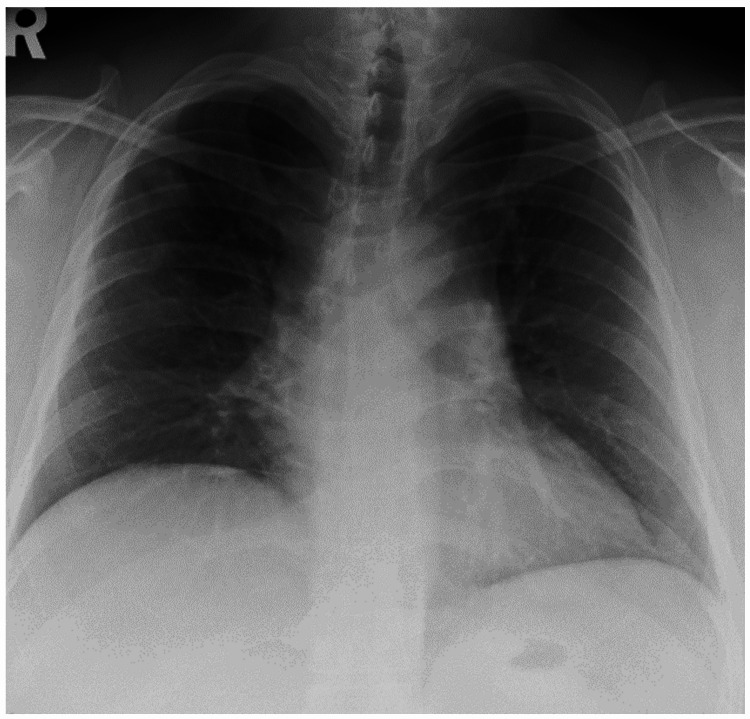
A chest radiograph obtained in January 2020 showed patchy shadows in the right upper lung field.
